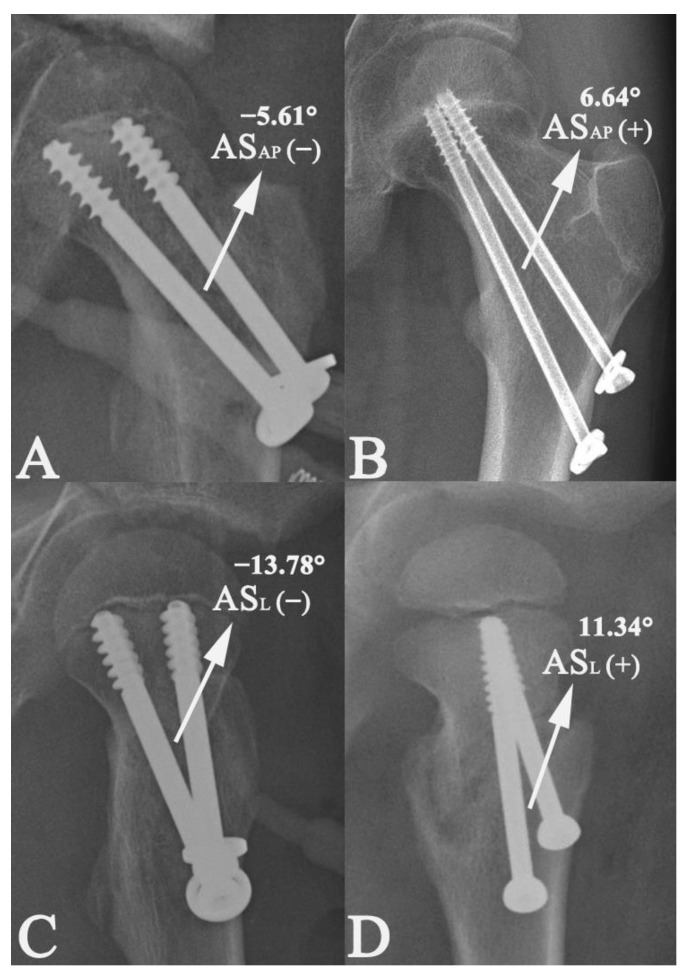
The angulation (AS) between the axis of two cannulated screws on anterior–posterior (AS_AP_) and lateral (AS_L_) radiographs were both measured and were expressed as degrees (°). The value of AS_AP_ or AS_L_ was defined as neutral (zero) if two screws were parallel, negative if two screws were divergent (˅-shape), and positive if two screws were convergent (˄-shape) (A–D).
